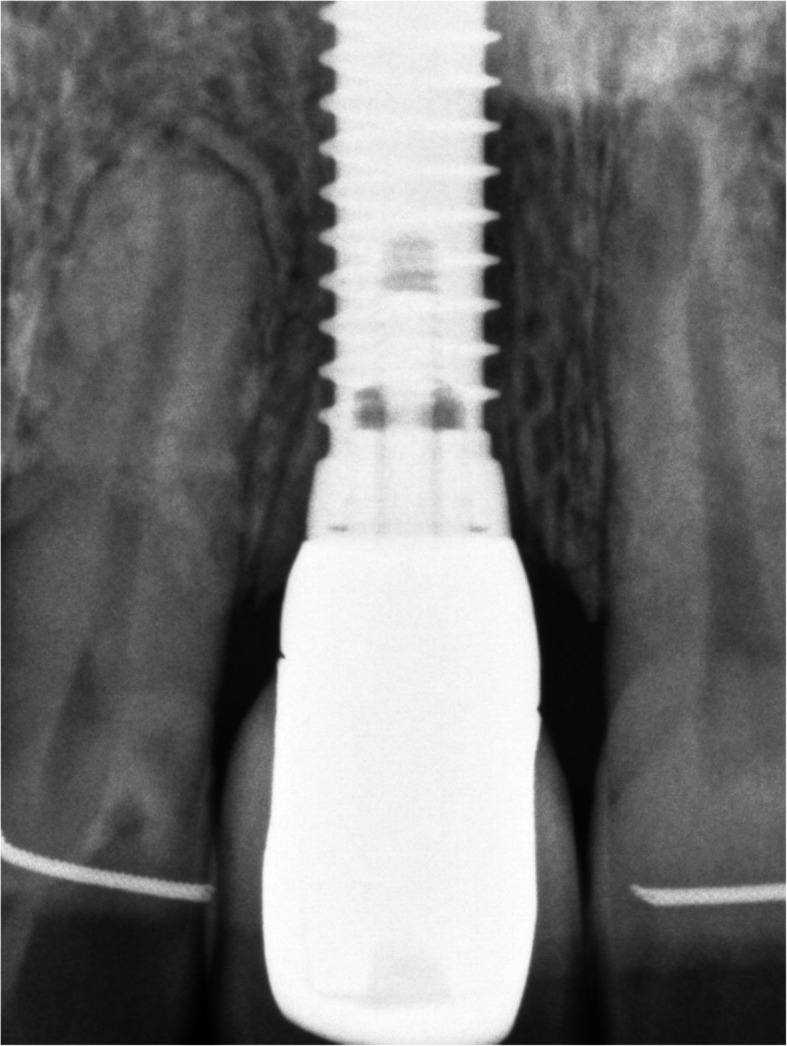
Postoperative x-ray at time of fitting of implant placement
